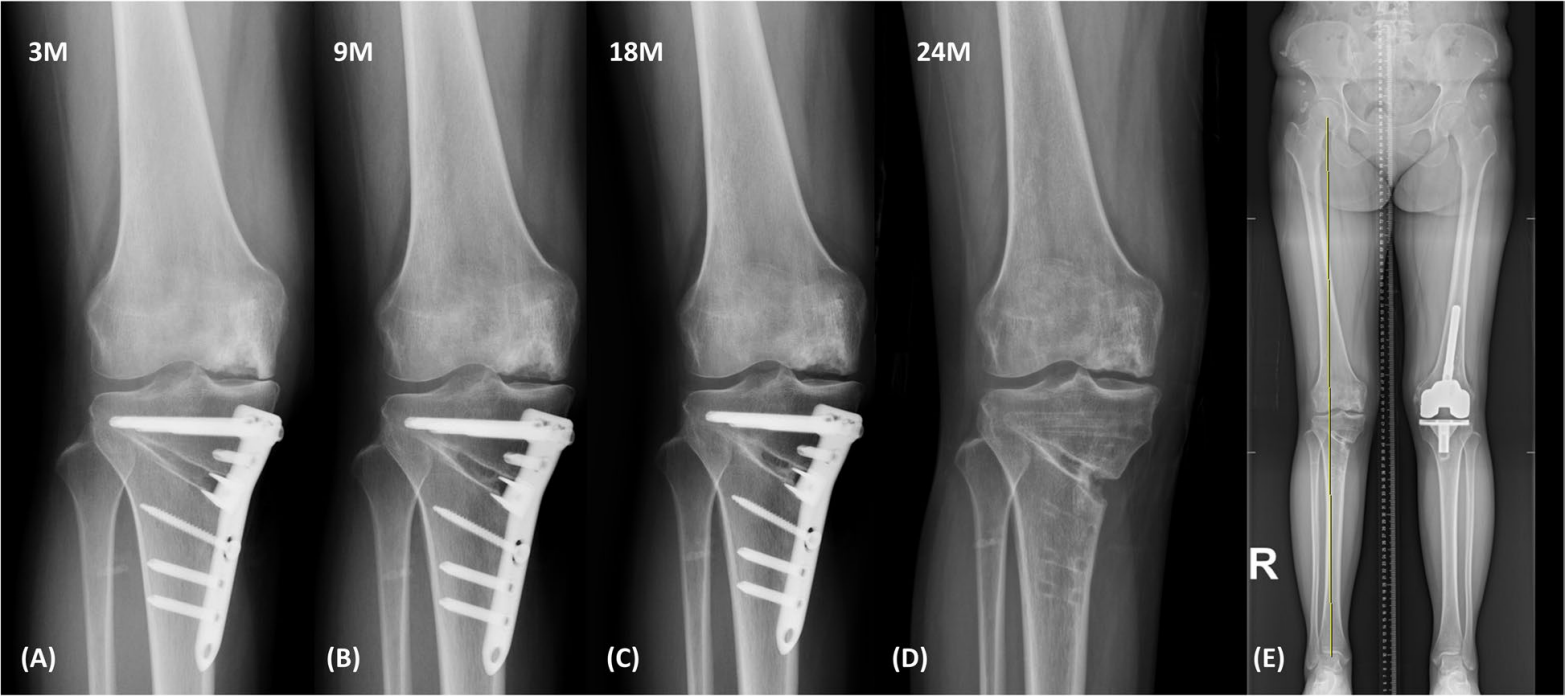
Standing anteroposterior radiograph of right knee after surgery: A 3 months, (B) 9 months, (C) 18 months, (D) 2 years. Progressive increase in medial joint space width is observed over time. Joint space width was 1 mm at 3 months, 1.5 mm at 9 months, 2.3 mm at 18 months, and 2.7 mm at 24 months postoperatively. E Scanogram of whole lower limb after metal plate removal at 2 years
